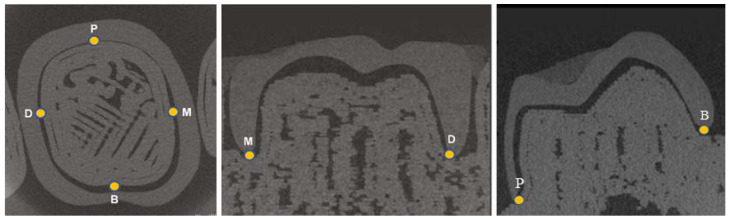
Provisional crown was placed on each model, and the marginal fit was measured using micro-computed tomography at the mesial center (M), distal center (D), buccal center (B), and palatal center (P) of the tooth.
